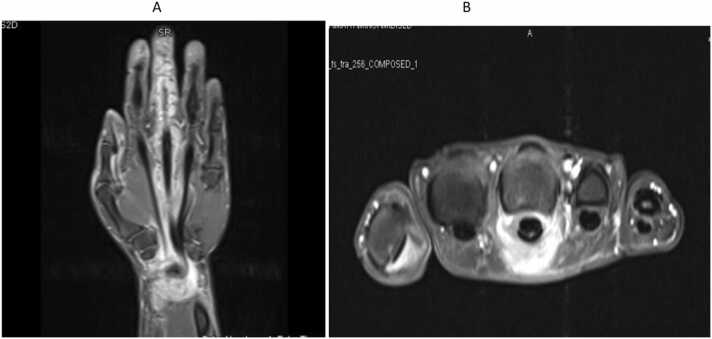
A and B MRI of the right hand demonstrating right middle finger tenosynovitis extending to the carpel tunnel.
